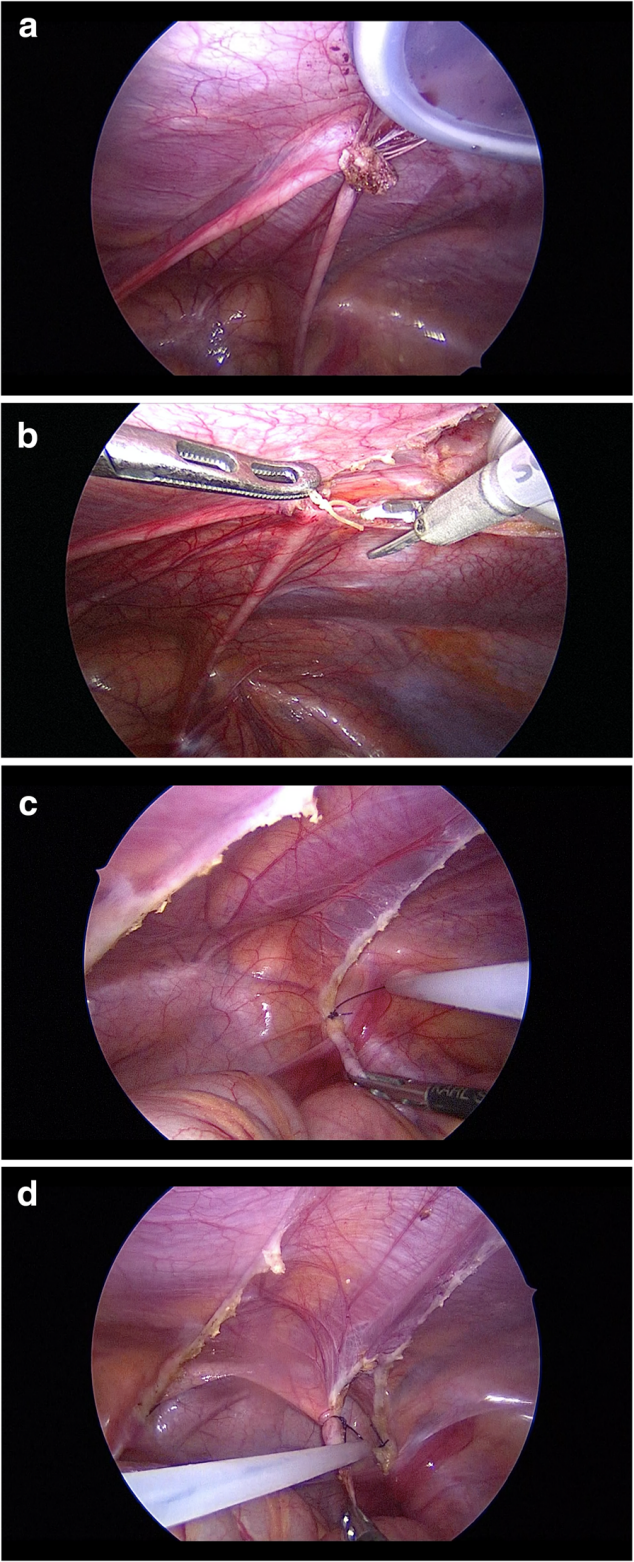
Laparoscopic view of the urachal remnant. a A wrap protector separated the cephalic side of the urachal remnant and the medial umbilical ligaments. b The urachal remnant was lysed off using ultrasonic scissors. c The caudal stump of the medial umbilical ligaments was tied with a single ENDOLOOP ligature®. d The caudal stump of the urachus was tied with a single ENDOLOOP ligature®
